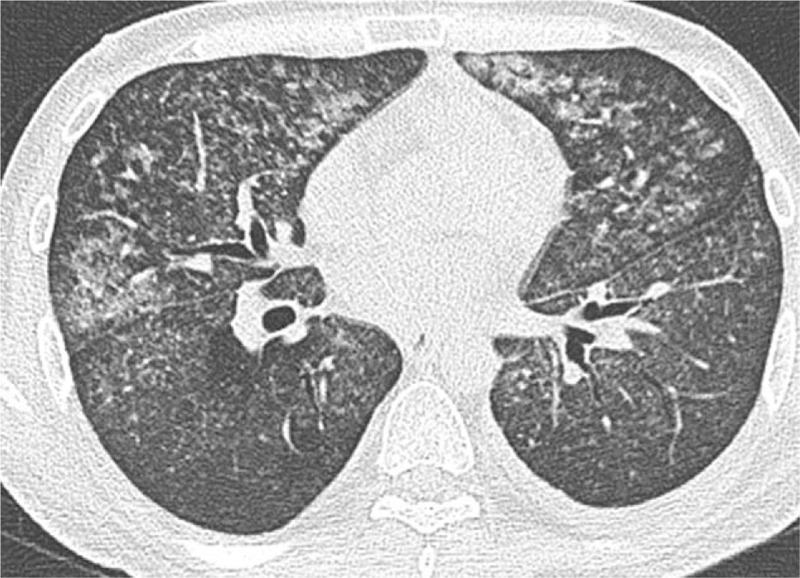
High-resolution CT scan showing diffuse multiple nodules in both lungs. Most nodules were 1 to 5 mm in diameter with smooth or irregular edges and were mostly located in the middle and inner lungs. Interlobular septal thickening, a small amount of left pleural effusion, and pleural thickening were observed.
